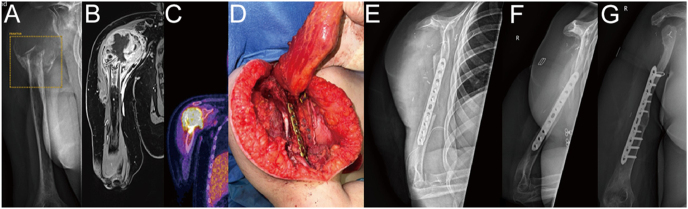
(A, B, C) Twelve-year-old girl with a high-grade osteosarcoma of the right proximal humerus (X-ray, MRI, and PET-CT); (D) intraoperatively; (E) 1 week postoperatively; (F and G) 1 year postoperatively.
